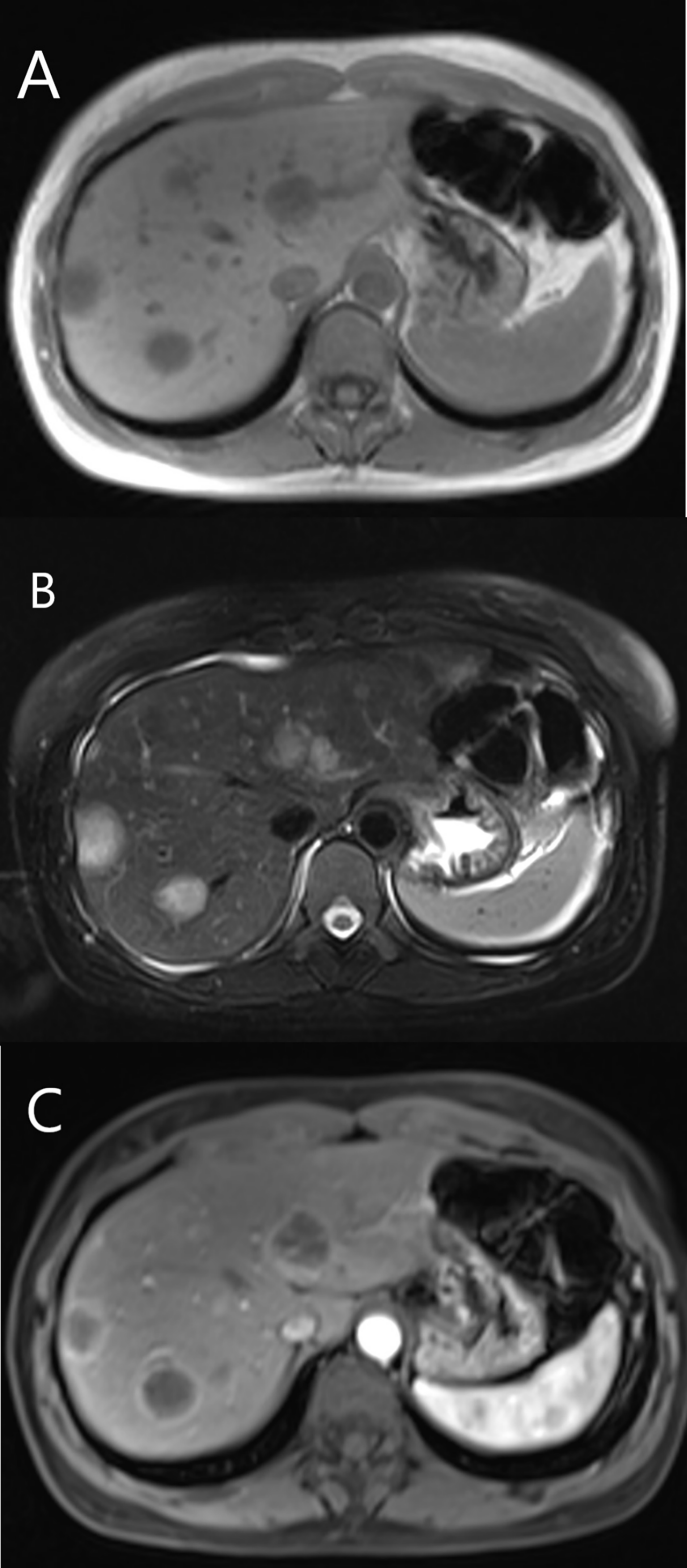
MRI features of HEHE in a 35-year-old female. (A) Hypointense showed on T1-weighted image. (B) The lesions displayed hyperintense on T2-weighted image. (C) Rim-like enhancement and lollipop sign were seen after contrast agent injection.
